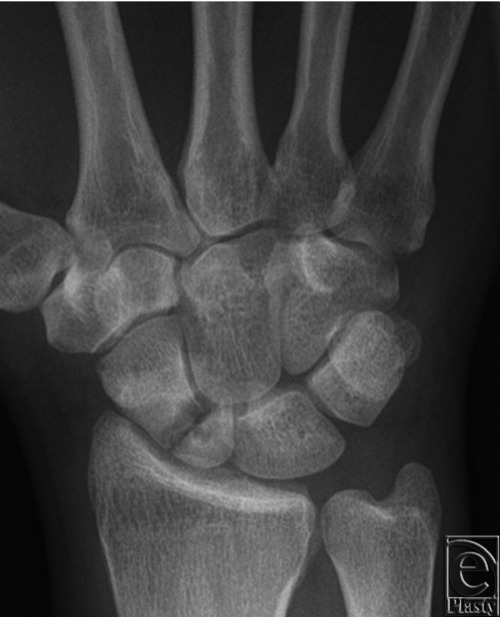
Posteroanterior radiograph showing a proximal third scaphoid nonunion.
